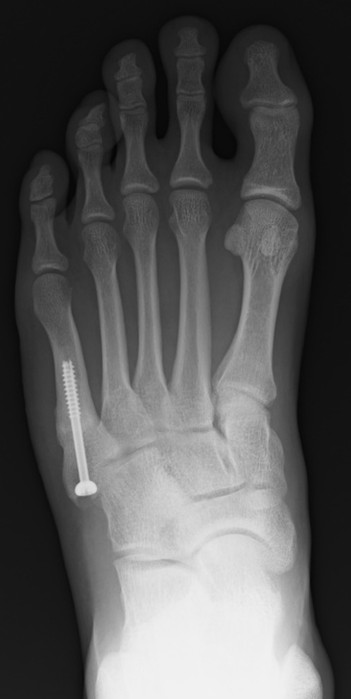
X-ray images from four directions to confirm bone union. Anteroposterior radiograph.
